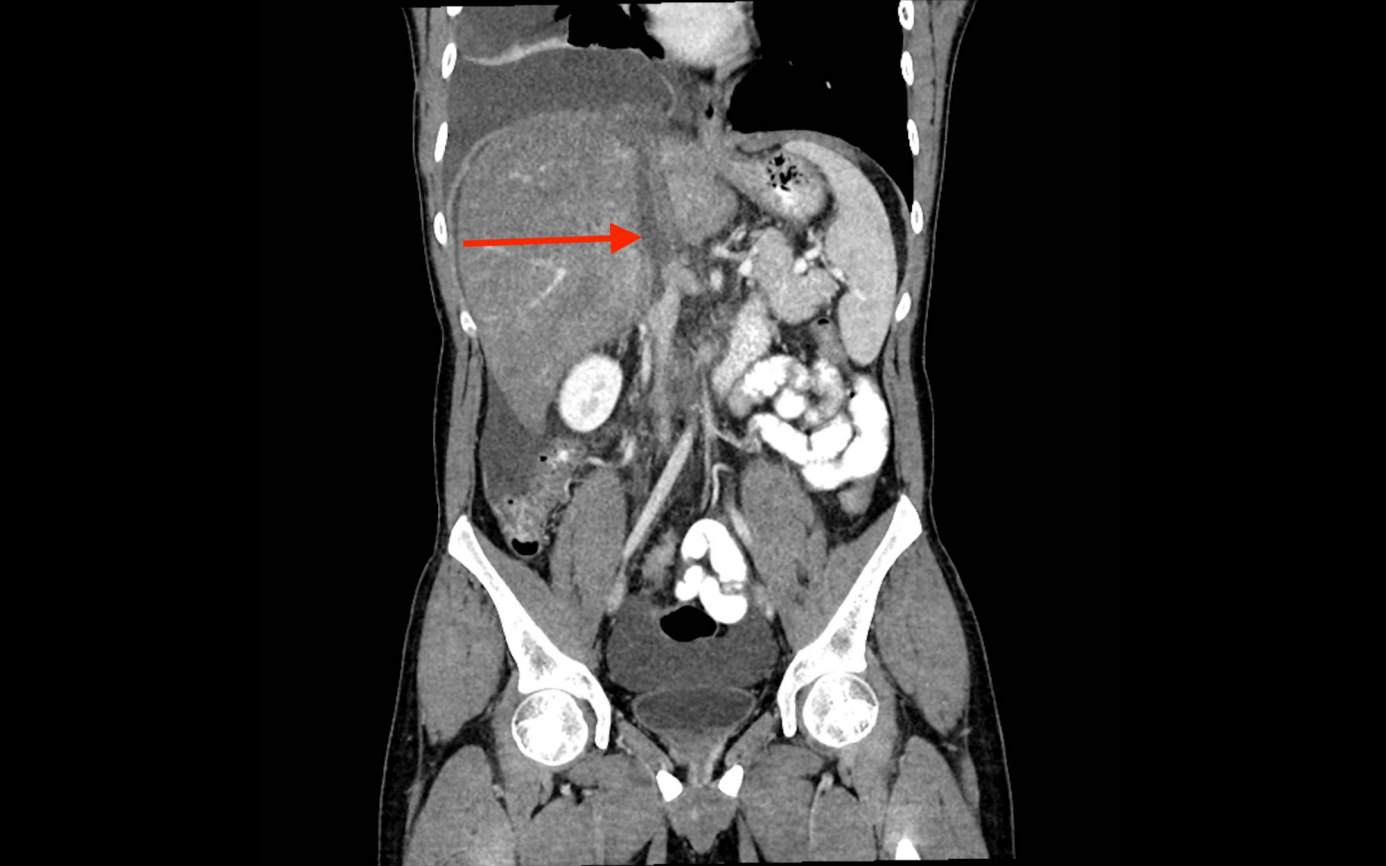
CT scan of the chest , abdomen, and pelvis with intravenous contrast , showing an IVC thrombosis. IVC, inferior vena cava
